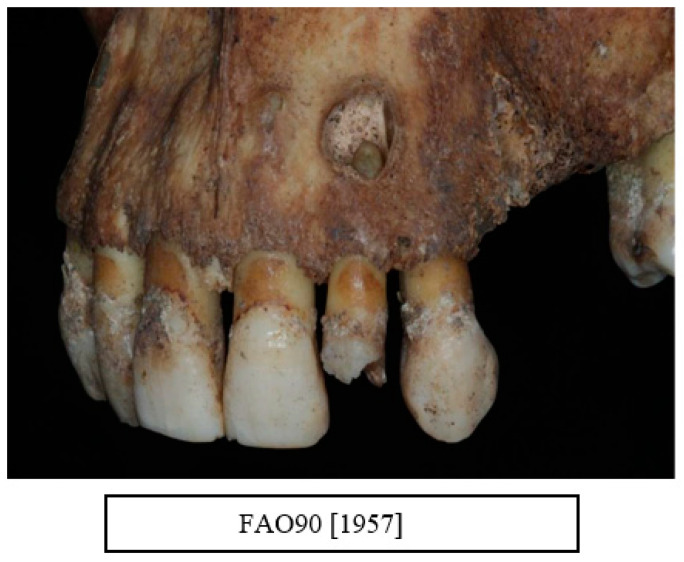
A periapical lesion associated with a retained root of an upper left second incisor tooth (UL2) (courtesy of the Museum of London).
